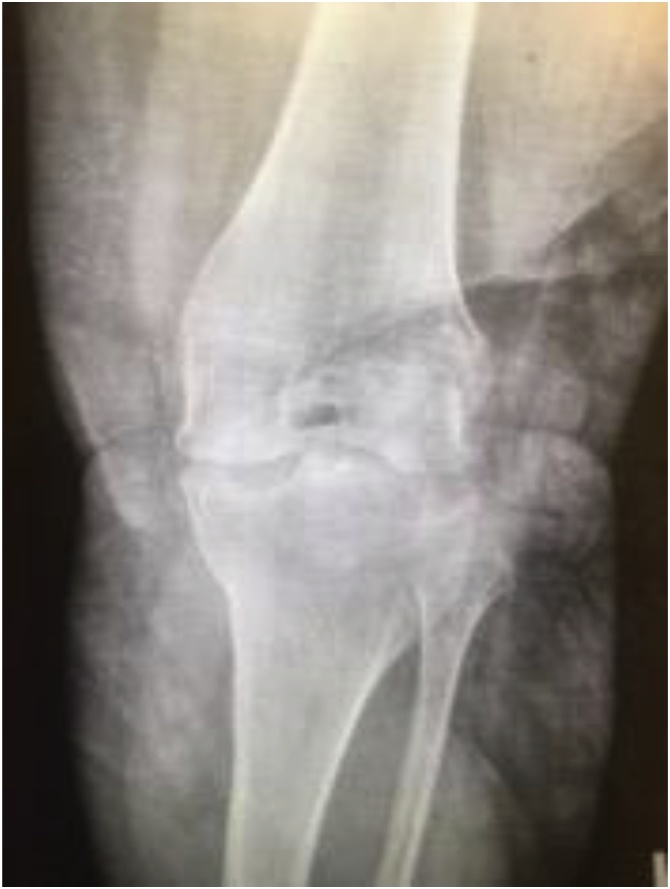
Left Knee AP Radiograph.
